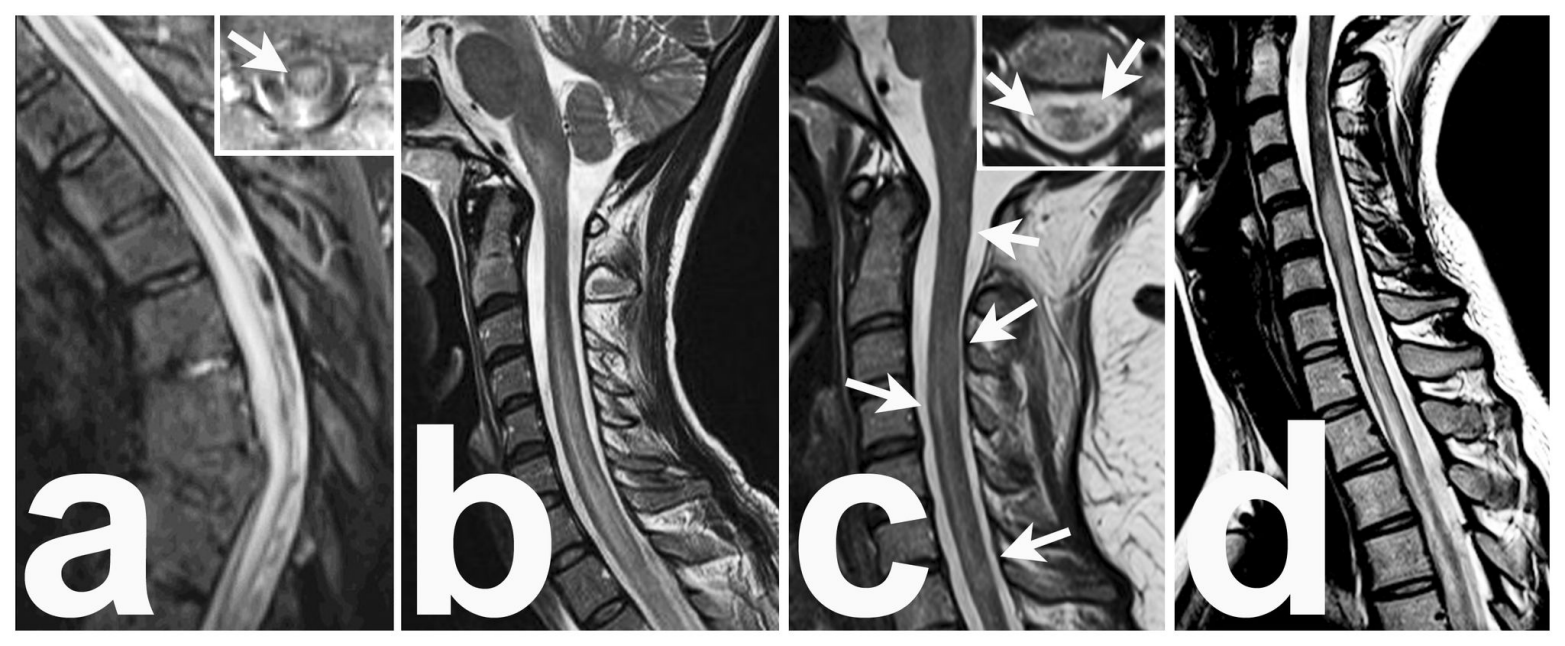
Differential diagnosis of NMO/NMO-SD associated LETM. a, a 90 year-old woman with left-sided chest pain, a three-day progressive paraparesis and Herpes simplex lesions gluteal. Inlay, the lesion involves the central regions of the spinal cord. CSF, 160 cells/μl (lymphocytes, (activated) lymphocytes, monocytes, neutrophils, eosinophils), no intrathecal Ig synthesis and no oligoclonal bands. Sera AQP4-ab negative. Diagnosis, HZV myelitis sine herpete (PCR from the vesicle contents, positive for HSV; PCR from CSF, negative for herpes simplex virus, but positive for varicella zoster virus.) . b, a 34 year old woman with peracute tetraparesis after infection of the upper respiratory tract and pneumonia. CSF, 65 cells/μl, elevated total protein and IgG concentration, positive OCB. Serum AQP4-ab were negative. Diagnosis, postinfectious myelitis (complement binding reaction and ELISA for mycoplasma pneumoniae were both positive). In a follow-up after 6 months the AQP4-ab were again negative. c, a 27 year-old woman with dysaesthesia and right-sided hemiparesis. MRI, several confluent lesions which are located at the lateral and posterior regions of the spinal cord. CSF, 9 cells/µl, intrathecal IgG synthesis and oligoclonal bands type 2b. Serum AQP4-ab were negative. Diagnosis, relapsing-remitting multiple sclerosis (Barkhof criteria fulfilled). D, NMO associated LETM, a 19 year-old women; CSF (3times), elevated cell counts, 9/µl, 181/µl and 207/µl, elevated total protein, but no IgG synthesis and no OCB. AQP4-ab were repeatedly positive (patient 71, Figure 2).
